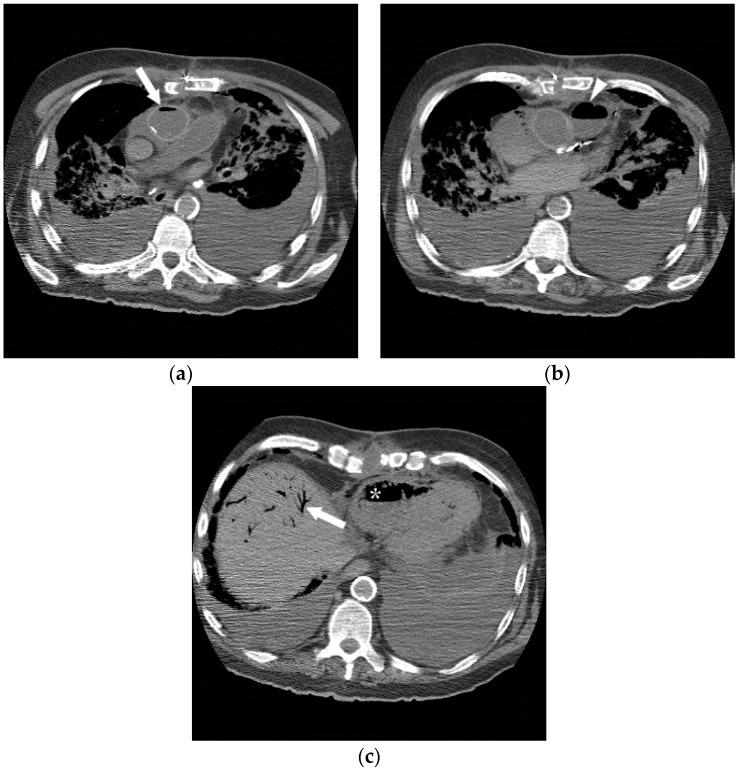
A 69-year-old man who was critically ill with sepsis, pneumonia, and Cerebral Vascular Accident (CVA). Immediately after his pulmonary arterial line was pulled, he developed sudden cardiopulmonary arrest, suspected to be related to air embolism. A post-mortem CT (a–c) was performed 18 h later, revealing intravascular air in the ascending aorta (a, arrow), pulmonary artery (b, arrowhead), right ventricle (c, *) and the liver (arrow). Given the extent of air, it was considered most likely that the air was related to post-mortem state. Despite these findings, even when the heart was opened under water at autopsy, no air escaped, highlighting the difficulty in diagnosis of air embolism in some cases.
